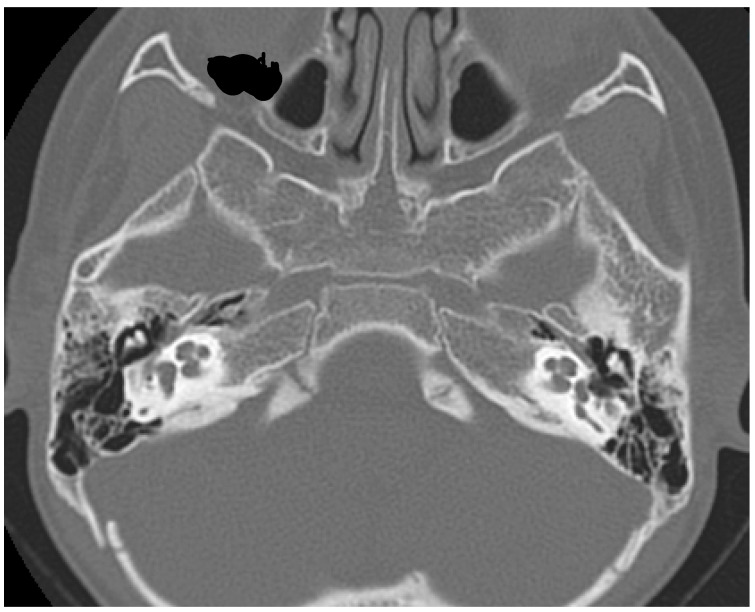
Petrous bone CT showing normal ear anatomy in axial view.
